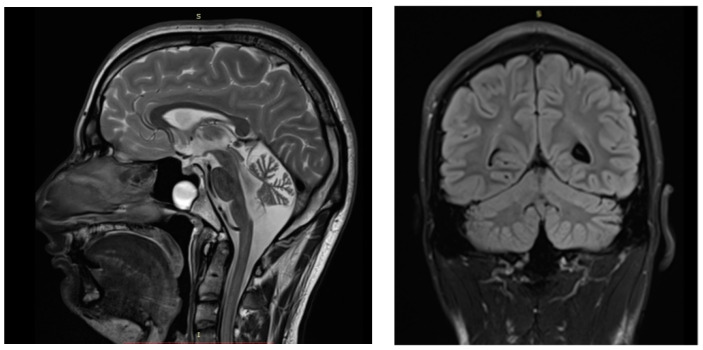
Brain MRI of the patient performed at 23 years. T1-weighted midsagittal section (left) and T2-weighted coronal (right).
